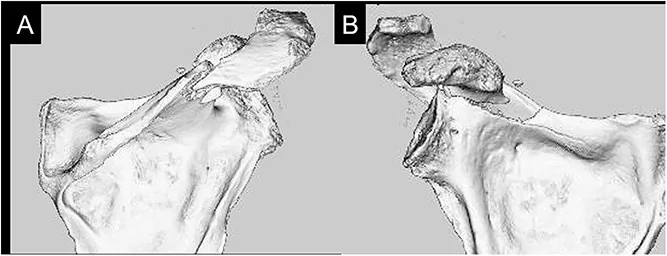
CT of the right scapula. 3D-CT demonstrates an Ogawa type III acromion fracture with a fracture line extending to the spinoglenoid notch (A) and an Ogawa type I coracoid process fracture (B).
